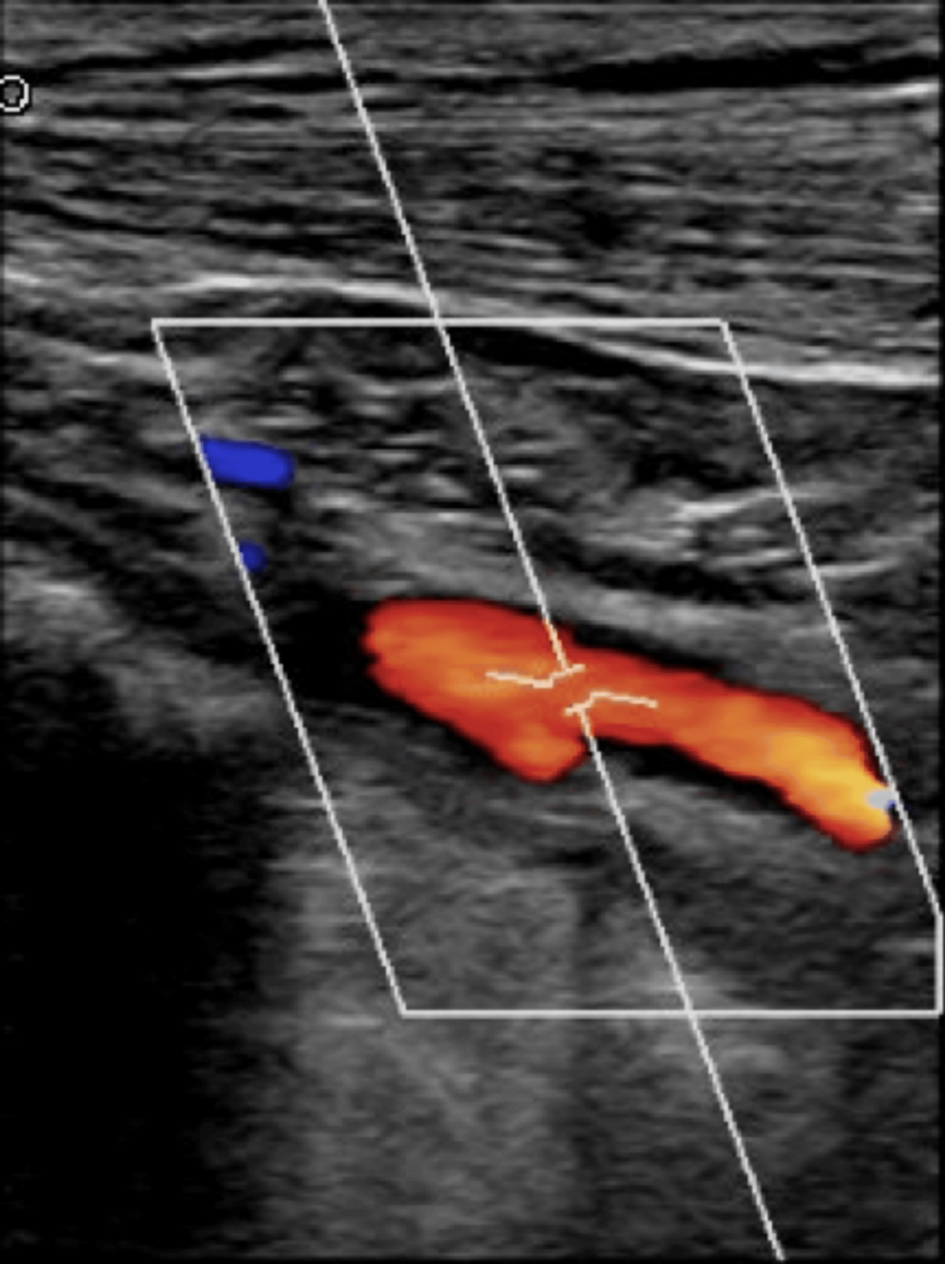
Subclavian artery with adequate flow on color Doppler assessment.
